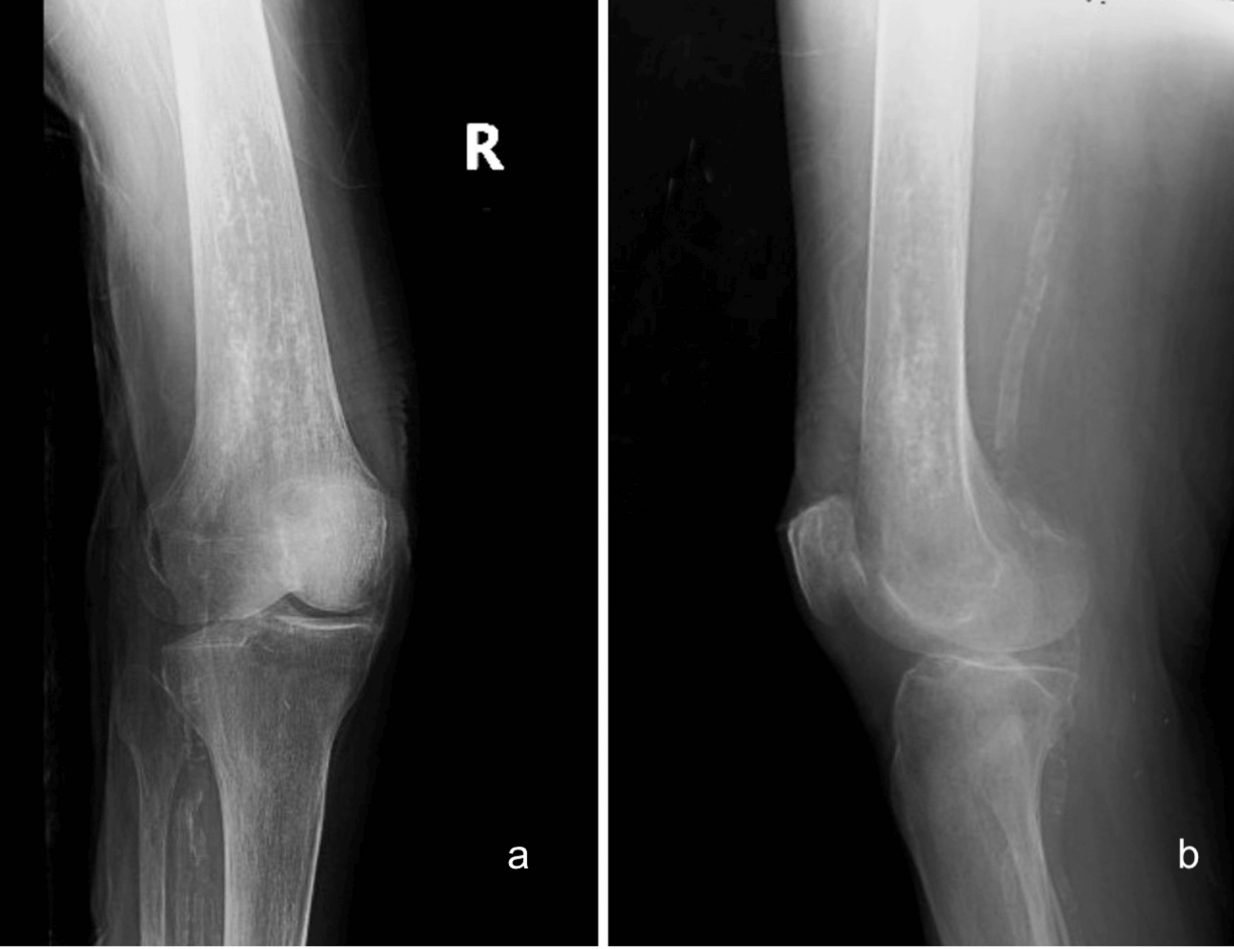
Anteroposterior (a) and lateral (b) radiographs of ipsilateral distal femur (right) showing intramedullary sclerotic lesion
